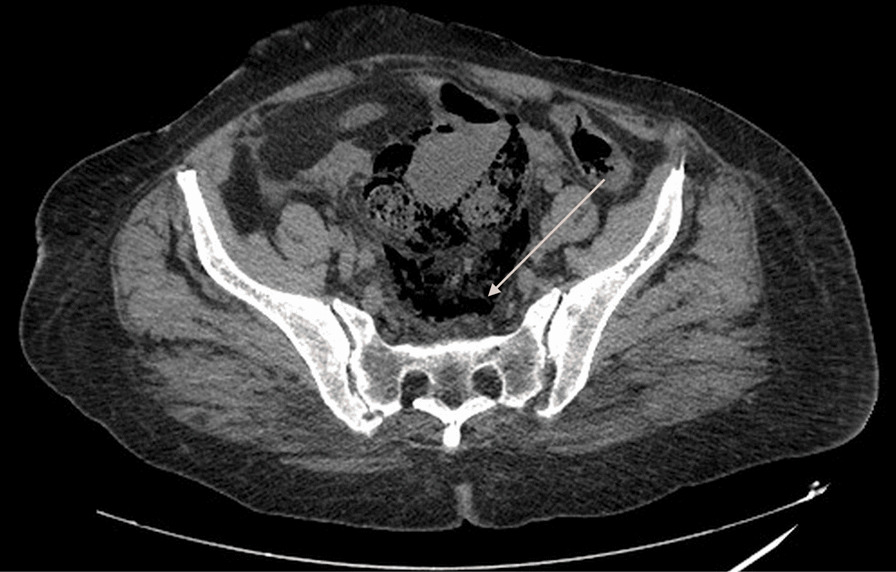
CT scan axial view showing pneumoretroperitoneum. Arrow points to pneumoretroperitoneum
